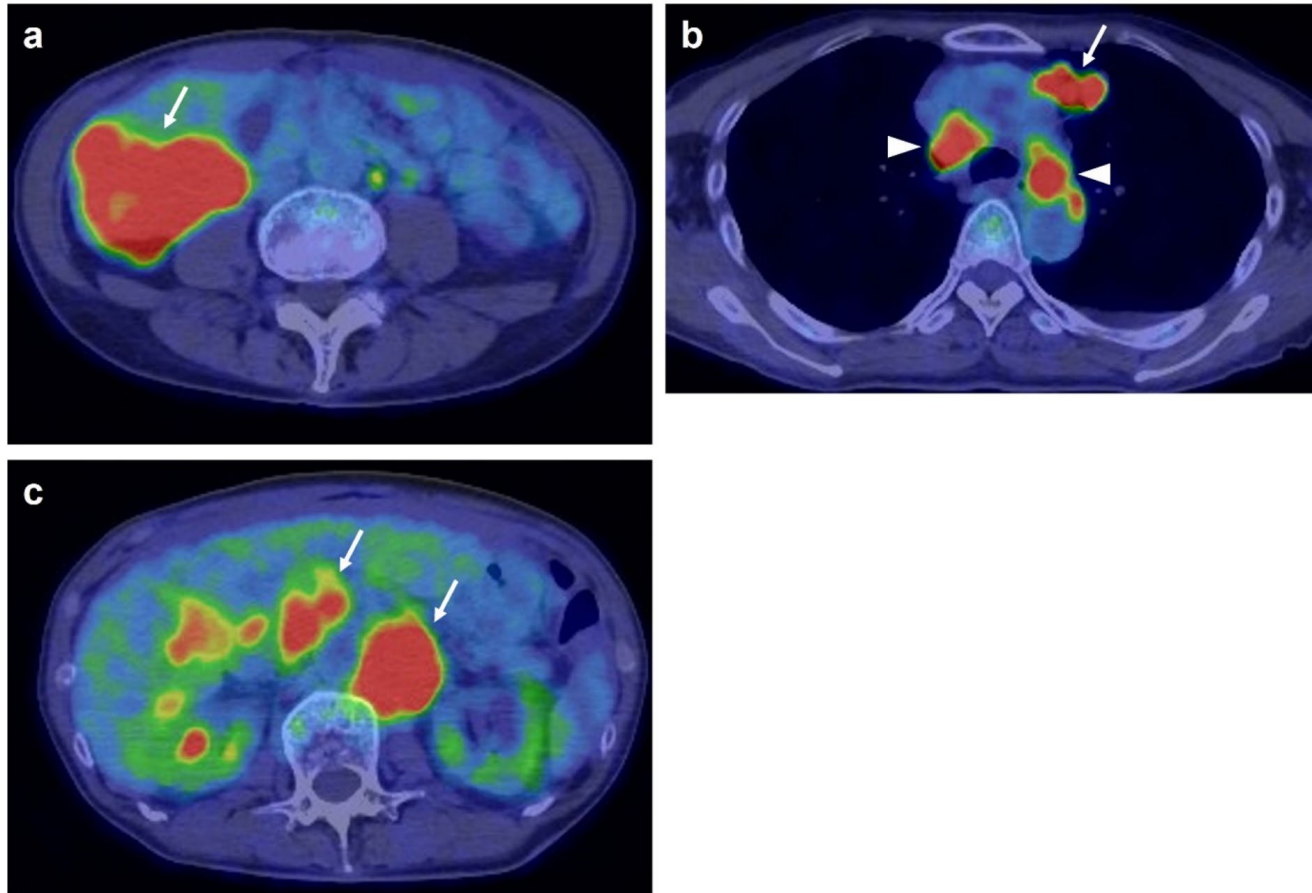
Whole-body positron emission tomography with 2-deoxy-2-[fluorine-18] fluoro-D-glucose combined with computed tomography (18F-FDG PET/CT). Hypermetabolic lesions in the liver (a: arrow), lungs (b: arrow), and lymph nodes (b: arrowheads; c: arrows) are observed
